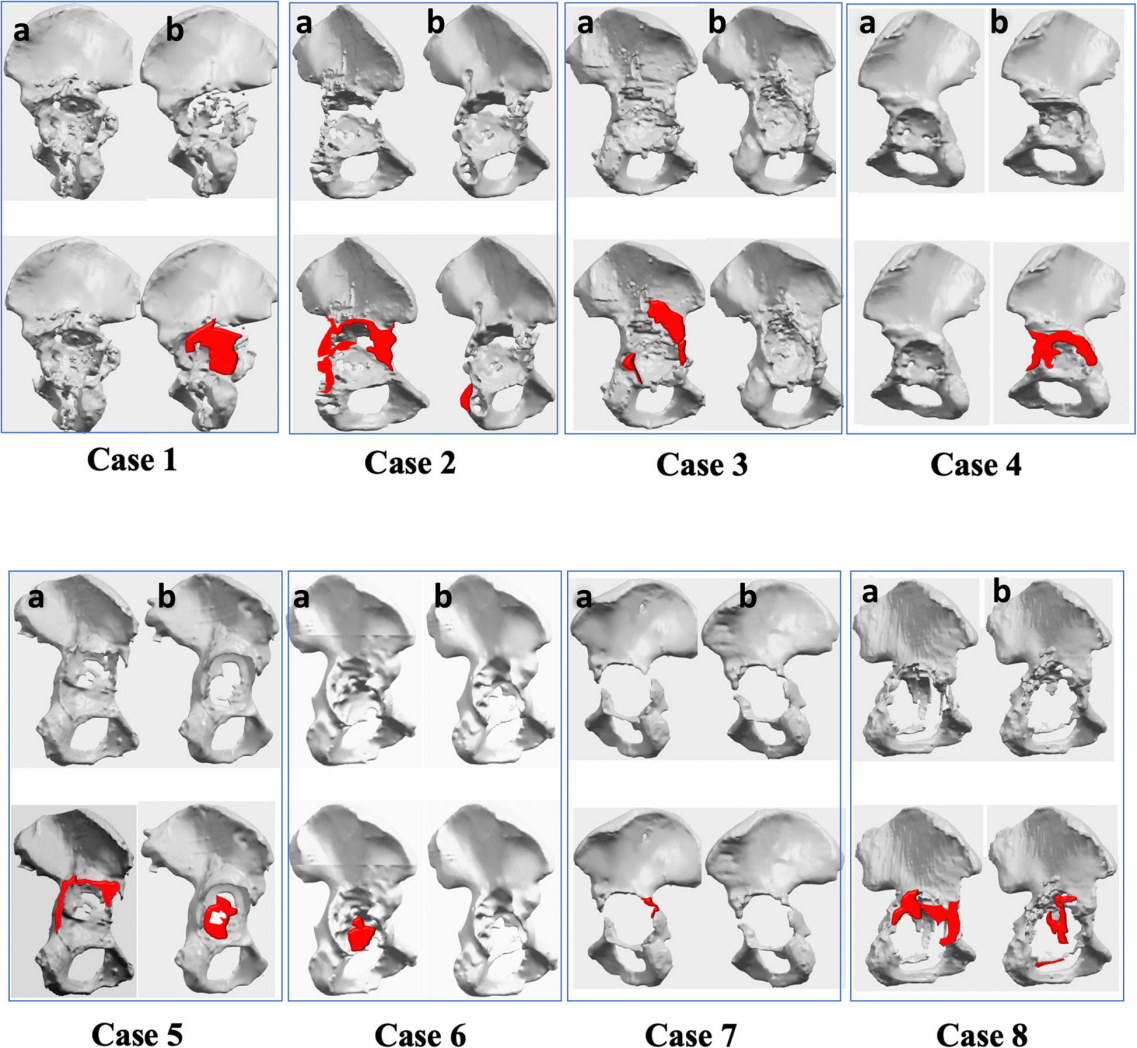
Pre and post implant removal reconstructed anatomies, a and b respectively. Parts in red represent the missing bone between the 2 CT reconstructions . Case 1: The volume of metal adjacent to the superior rim (cage, screws and femoral head) dramatically affected the true bony anatomy of the superior rim which resulted in a major change in the design of the 3D printed implant. Cases 2, 3, 5 and 6: images a showed reduced bone in the superior acetabular rim, b showed absent medial acetabular wall due to bone removed at surgery or not clearly visible on pre-removal scan. Case 4: the anterior wall was absent in the post-implant removal CT reconstruction (b). For case 7 and 8, the acetabular implant was loose and migrated into the pelvis and away from the innominate bone. Minimal differences between pre and post implant removal scans were seen
